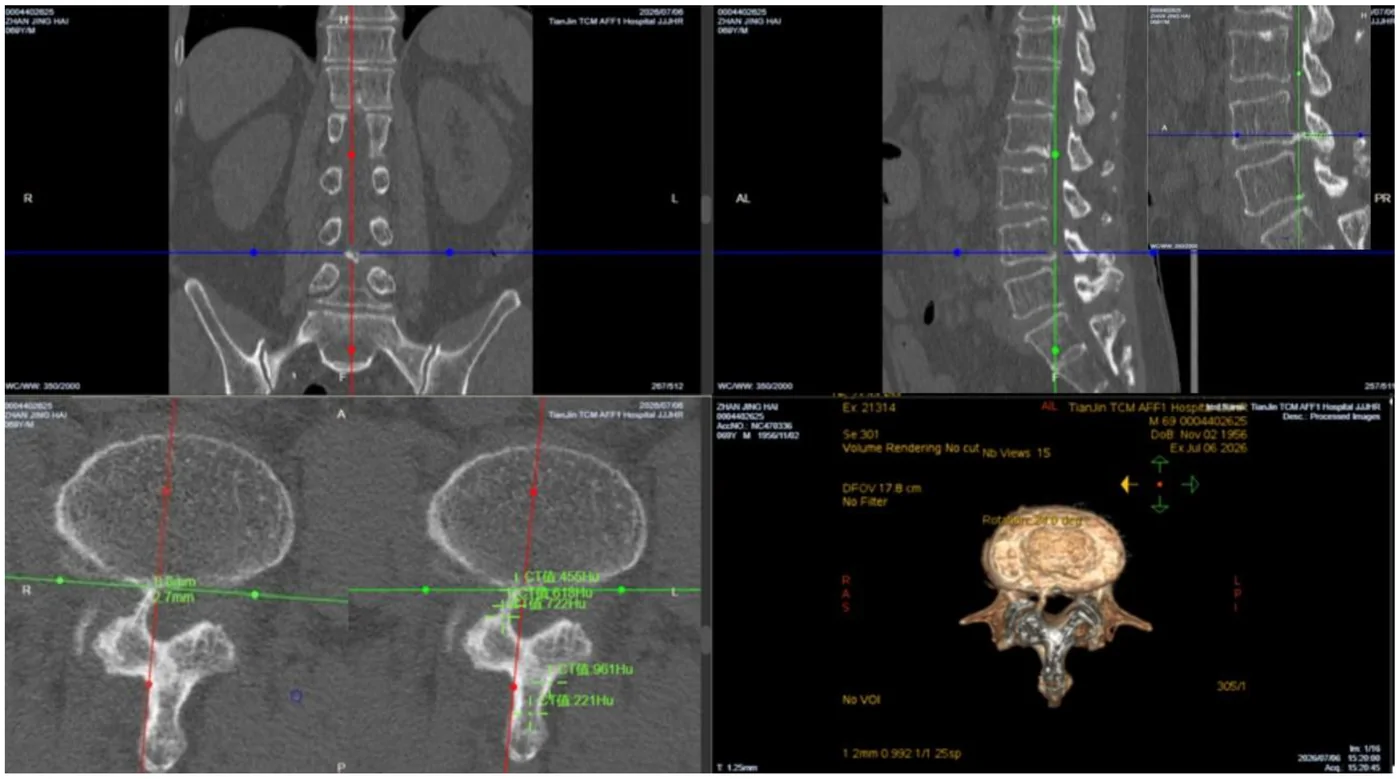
Imaging findings approximately 2 years after surgery. The left axial image shows the measurement of the bridging structure at the corresponding anatomical level, while the right sagittal image shows the slice with the greatest vertical extent. Bone regrowth was observed at the L4 laminectomy defect, forming a continuous bridging bony structure with the posterior margin of the L4 vertebral body. The CT attenuation values were 455 HU, 618 HU, and 722 HU at the ventral, middle, and dorsal portions, respectively, with a mean value of 598 HU. This value was intermediate between the cortical bone (961 HU) and cancellous bone (221 HU) of the contralateral spinous process at the same level, supporting the bony nature of the bridging structure. The structure measured approximately 8.8 mm in the anteroposterior dimension, 2.7 mm in the transverse dimension, and 4.4 mm in height.
